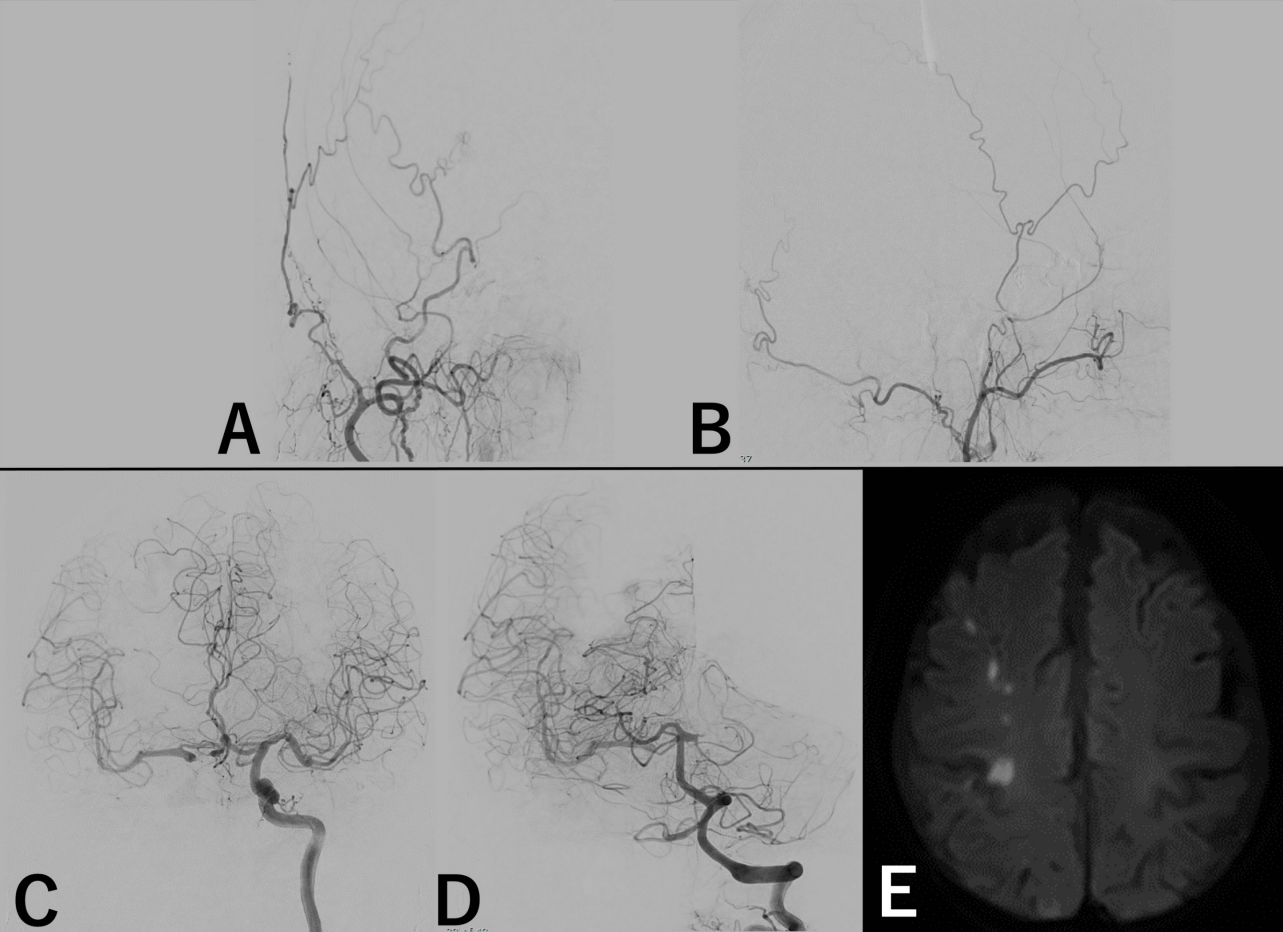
Images after embolization. (A) Right common carotid angiogram anterior-posterior view. (B) Right common carotid angiogram, lateral view. (C) Left internal carotid angiogram, anterior-posterior view. (D) Left vertebral artery angiogram, lateral view. (E) Postoperative head MRI (diffusion-weighted imaging).
